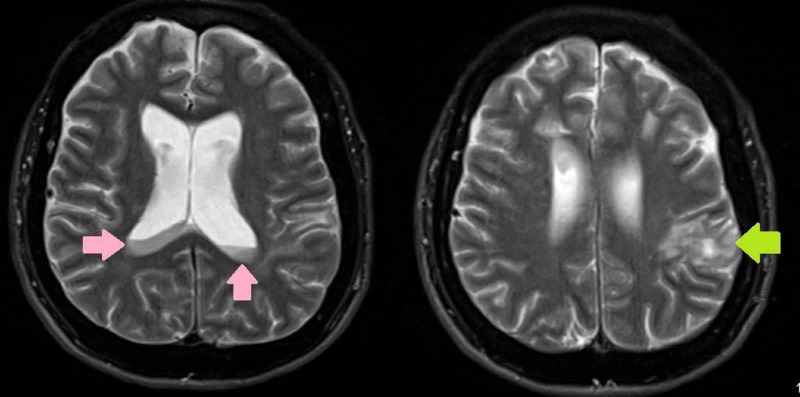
Magnetic resonance imaging of the brain showing pus in the ventricles (pink arrows) and a developing abscess in the left parietal lobe (green arrow)
